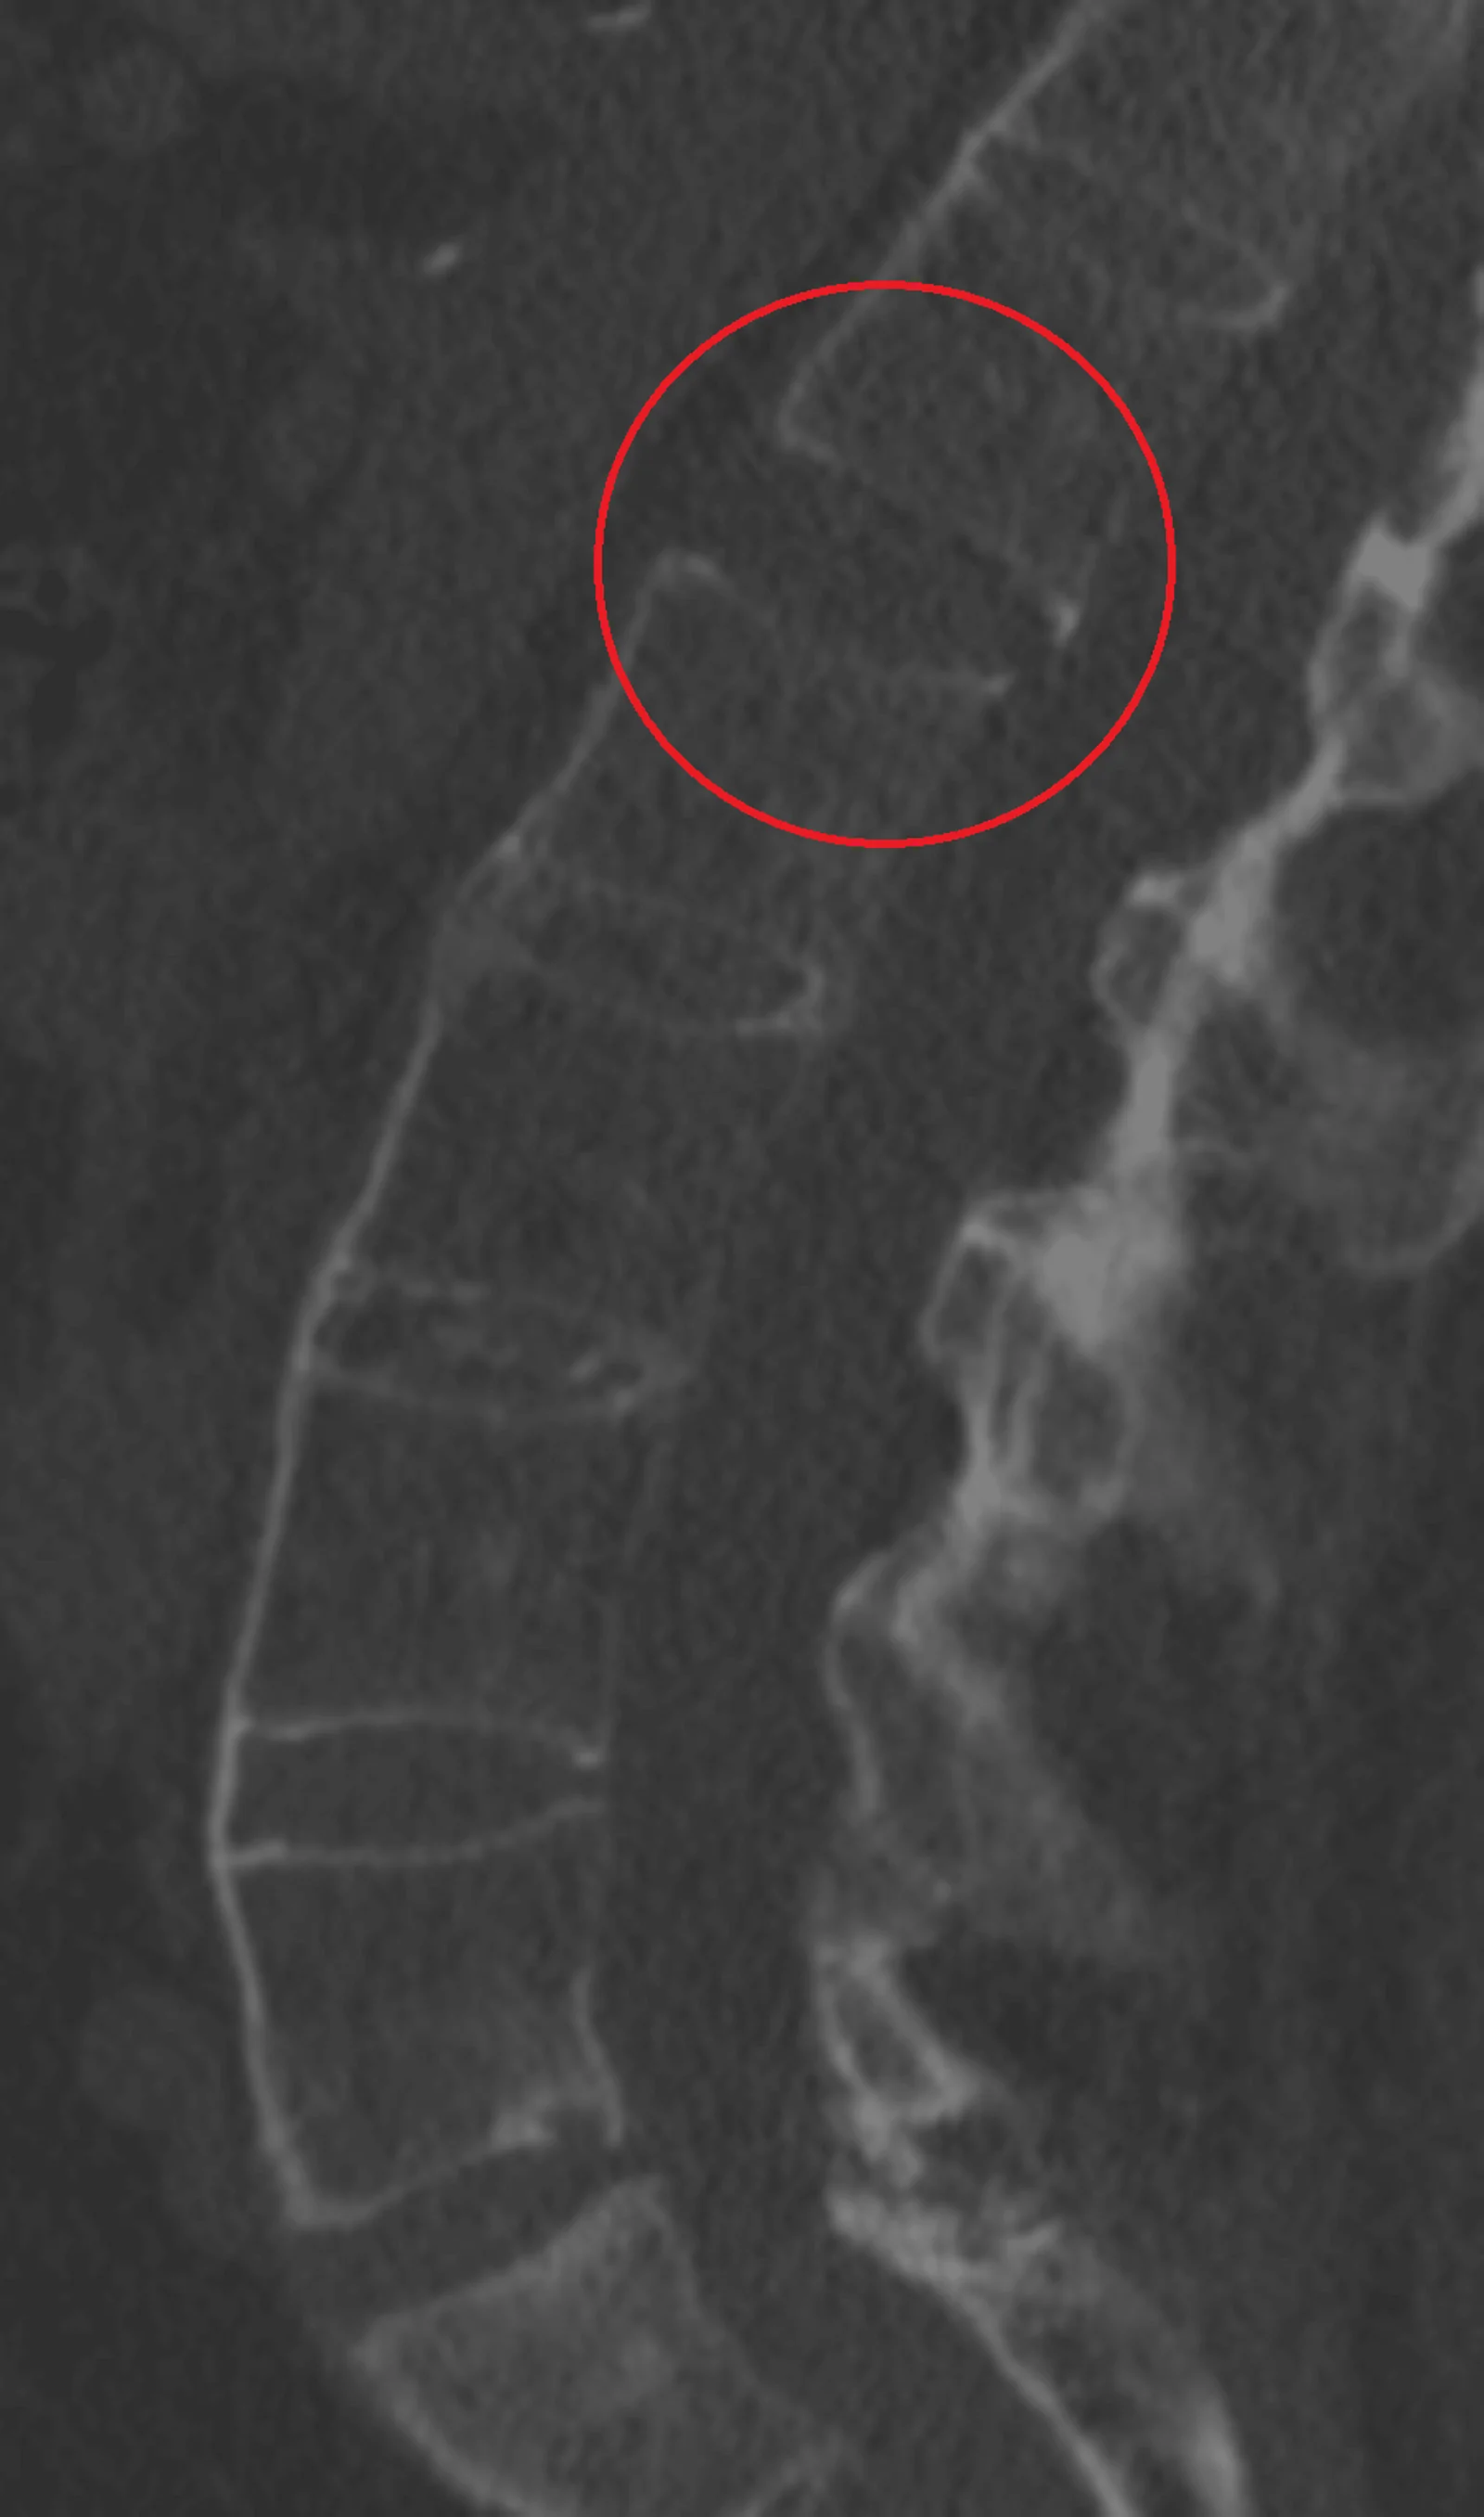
Sagittal CT image demonstrating marked widening of the L1-L2 intervertebral disc space with a transverse fracture extending through the ankylosed spine.
